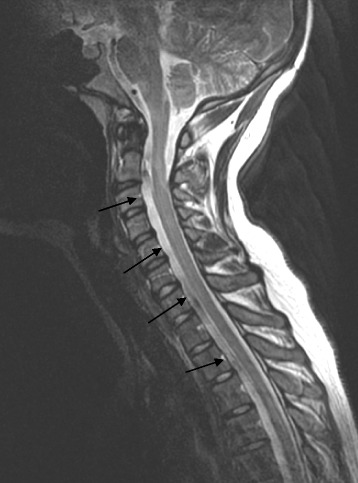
T2-weighted MRI of the spinal canal in the sagittal plane demonstrates a probable arachnoid cyst located anterior to the spinal cord from C2 and downwards. Additional MRI of the thoracic spine showed extension down to Th10. The MRI was performed one year after the accident.
